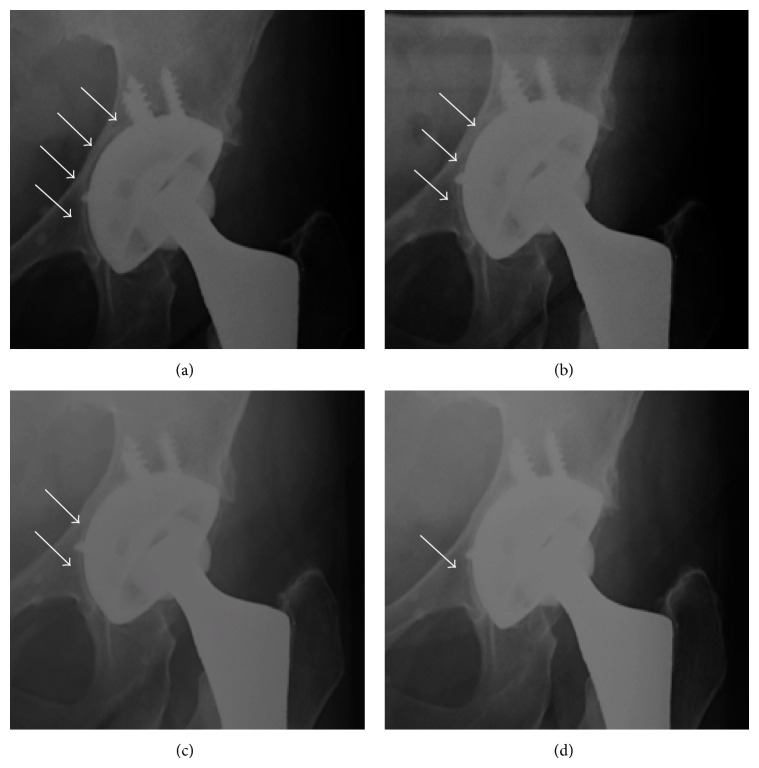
(a) Postoperative radiographs showing radiolucent lines which involved two adjacent sectors of the cup surface. ((b), (c), and (d)) Progressive osseointegration during 3, 6, and 12 months' follow-up.
